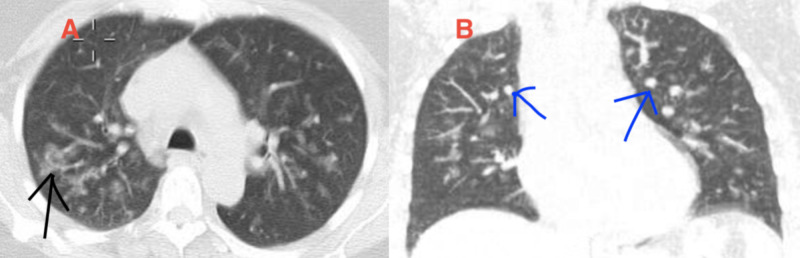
Multifocal nodular airspace opacities seen on chest CT Sub-panel A: axial view showing multifocal opacities (black arrow); Sub-panel B: coronal view showing multifocal nodular opacities (blue arrows) CT: computed tomography
